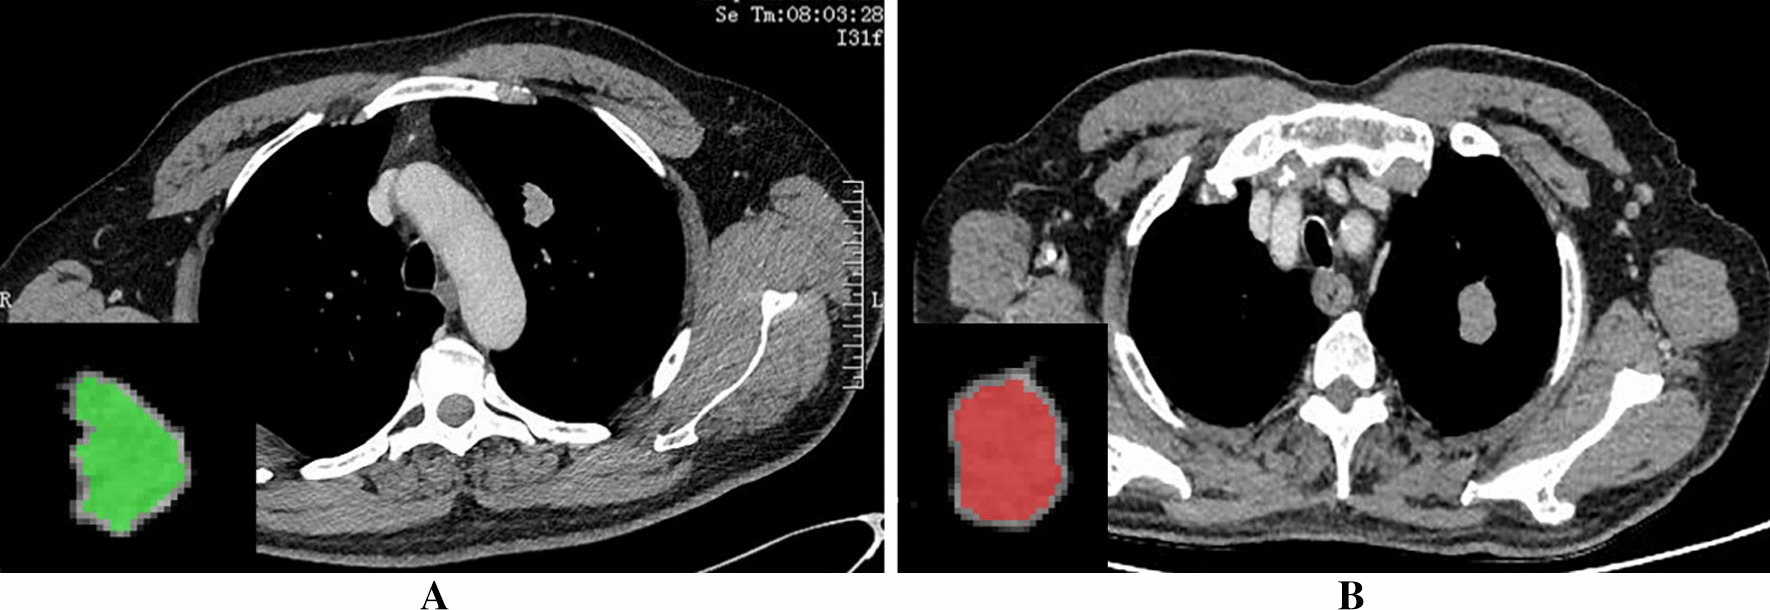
A 50-year-old man with a diagnosis of PSP (A). CT image of venous phase showed a left pulmonary nodule. The nodule is about 2.2 cm in length and diameter, with obvious uniform enhancement and lobulation sign at the edge. Manually outline the ROI 1-2 mm along the inner side of the nodule edge, which is indicated by the green area. A 76-year-old man with a diagnosis of Peripheral lung cancer (B). The CT image of the venous phase showed a left pulmonary nodule with a length of about 2.3 cm, moderately and uniformly enhanced, with smooth edges and lobular signs. Draw the ROI manually 1–2 mm along the inner side of the nodule edge, indicated by the red area
